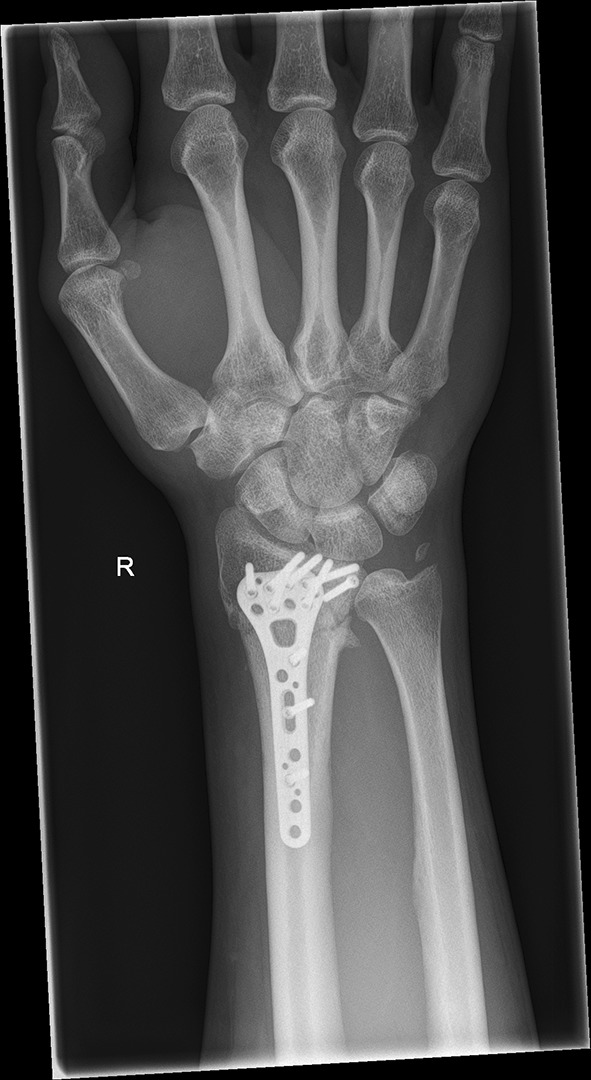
Dorsovolar and lateral x-ray control four weeks after surgery
